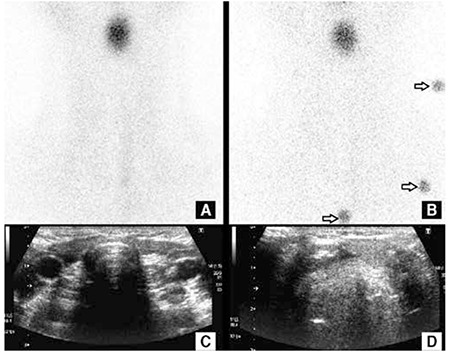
Thyroid scintigraphy (A, B) and US (C, D) of a 13 year-old-boy with hypothyroidism. Thyroid scintigraphy revealed focal radioactivity due to an ectopic gland, in the midline in the upper neck. Tc-99m O4 activity was not observed in thyroid area. US confirmed the diagnosis and revealed the ectopic thyroid gland below the hyoid bone in the midline
